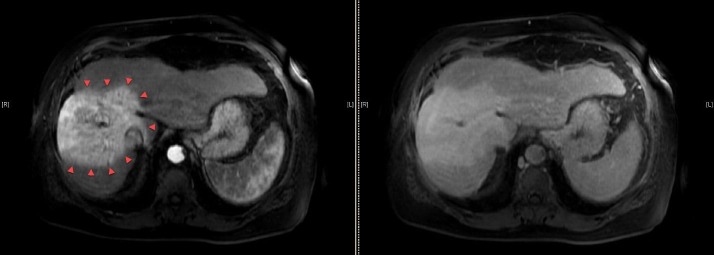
Post-radiation diagnostic magnetic resonance imaging (MRI) of the abdominal scan. The MRI abdominal scan axial images seven months after completion of proton therapy. Areas of hyperenhancement (red arrowheads) on arterial phase (left panel) correspond to planned dose distribution shown in Figure 3. No areas of washout are seen on delay phase (right panel), signifying a complete radiographic response.
